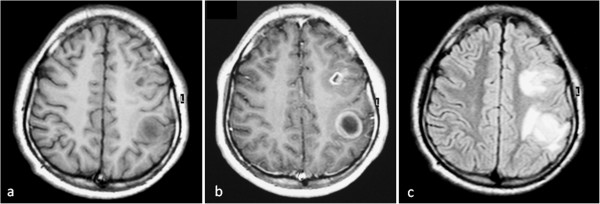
Brain MRI showing reduction of the size of the cysts and perilesional edema after receiving Albendazole. Pre (a), Post contrast (b), T1WI and FLAIR (c) showing reduction of the size of the lesions and perilesional oedema after 3 months of treatment. The size of the largest lesion is 2 cm in diameter.
